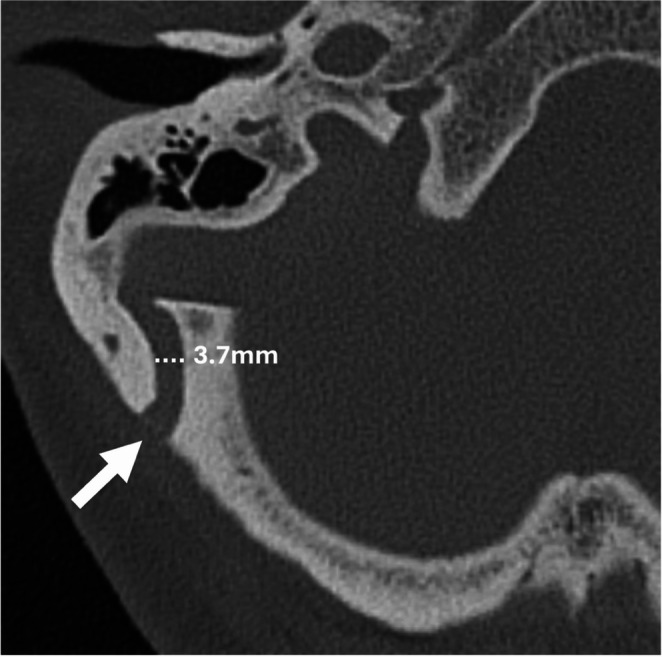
Enlarged mastoid emissary vein. Axial CT demonstrating an enlarged mastoid emissary vein (arrow) connecting the sigmoid sinus with extracranial veins, which should be recognised to avoid inadvertent injury
